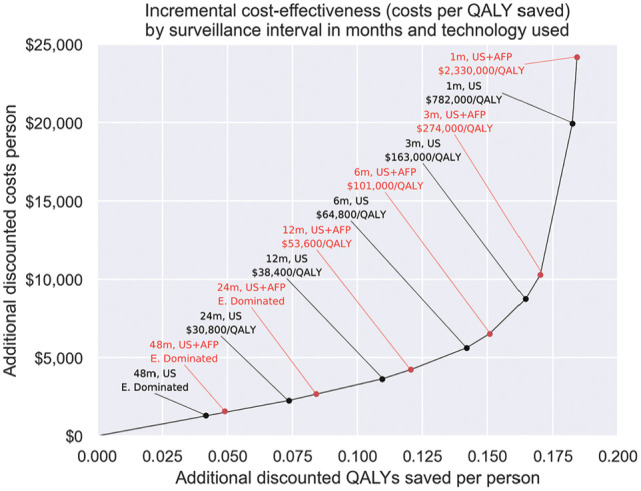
Discounted costs versus discounted QALYs saved for routine US surveillance with or without AFP by surveillance interval in months. Scenarios not on the cost-effectiveness frontier are extended dominated and marked as E. Dominated. AFP, alpha-fetoprotein; QALY, quality-adjusted life-year; US, ultrasound.
